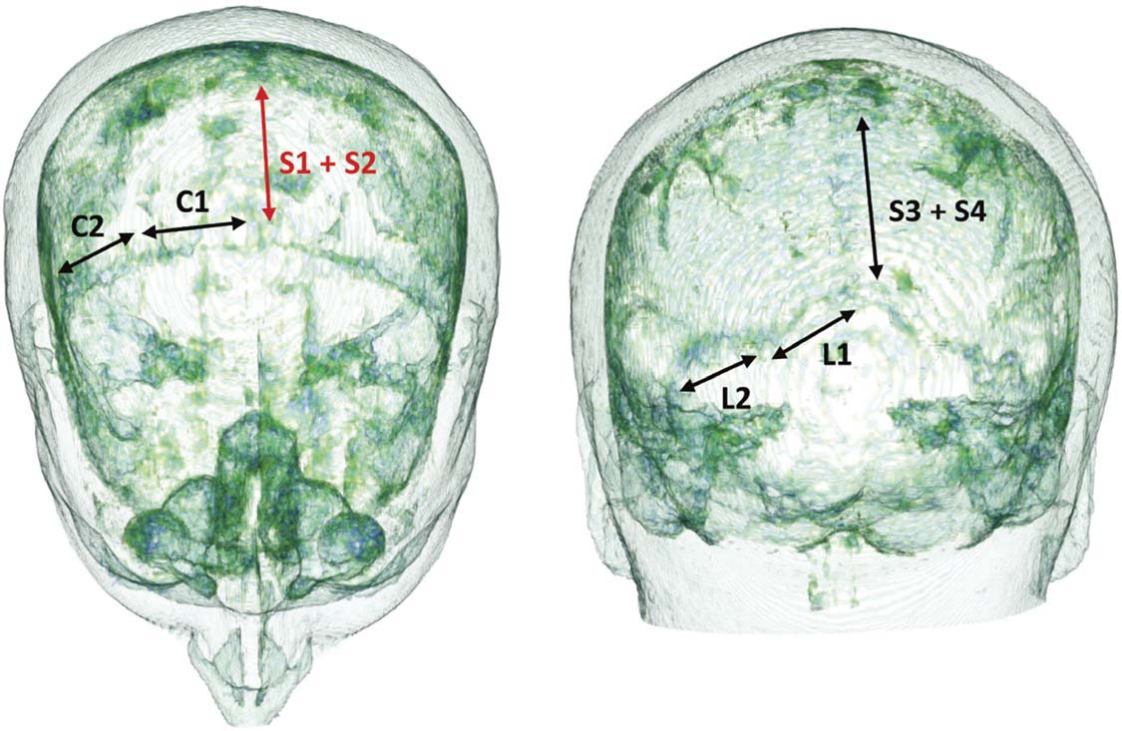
Volumetric rendering of T1 MRI images—DICOM images from same subject (AC) used in the ROAST models uploaded to “3D Slicer 5.0.2.” Volume rendering was shift adjusted so the skull and sutures were visible using the preset “CT-Fat.” C1 and C2 represent the coronal suture: first part and second part. S1, S2, S3, and S4 represent 4 parts of the sagittal suture and S1 and S2 have closed and not visible represented in red. L1 and L2 represent the lambdoid suture: first part and second part.
